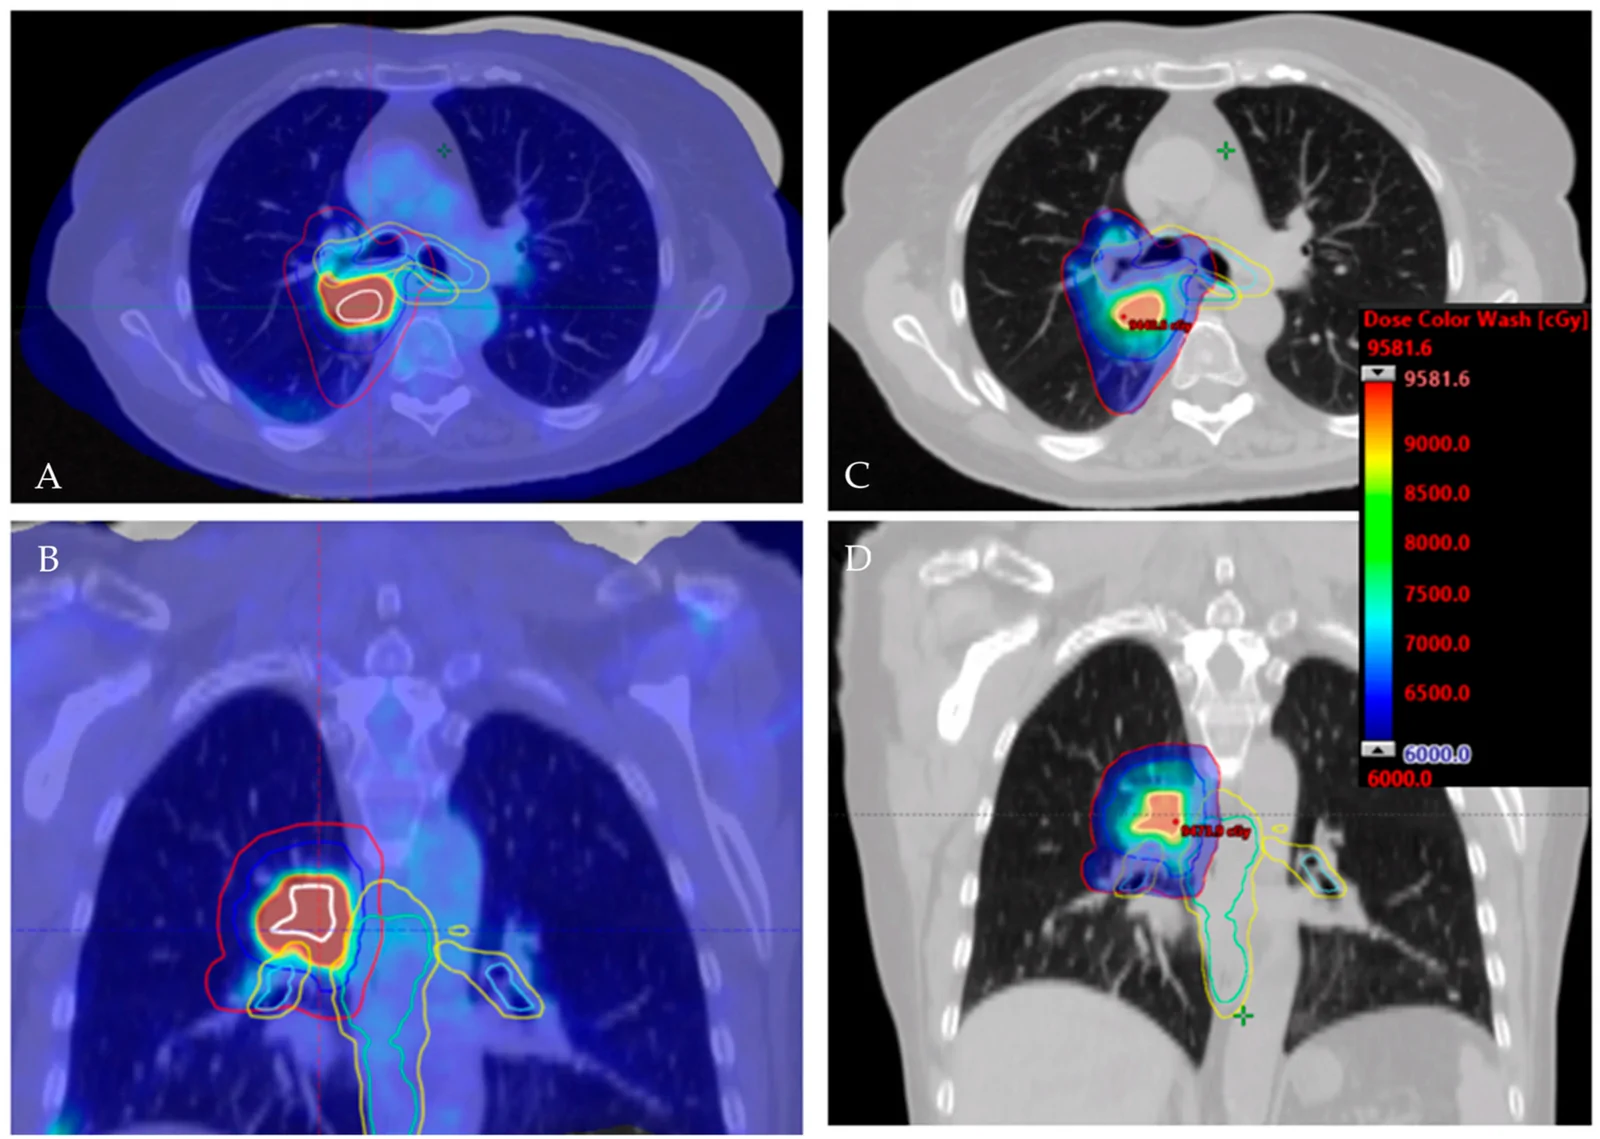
(A,B) Right lower lobe, unresectable LA-NSCLC, demonstrated on non-contrast four-dimensional radiotherapy planning CT. Co-registered PET-FDG in planning position is superimposed. Red line: planning tumor volume (PTV); blue line: clinical target volume (CTV); white line: PET-FDG-based dose-escalated subvolume (50% of SUVmax was chosen as the threshold for volume generation); cyan line: main bronchi; green line: esophagus; yellow line; planning organ-at-risk volumes of esophagus and bronchi. (C,D) Treatment plan with dose superimposed. Volumetric arc therapy (VMAT) was the technique of choice. Through simultaneous integrated boost (SIB), 60 Gy, 66 Gy and 90 Gy were prescribed to PTV, CTV and PET-FDG subvolumes, respectively.
